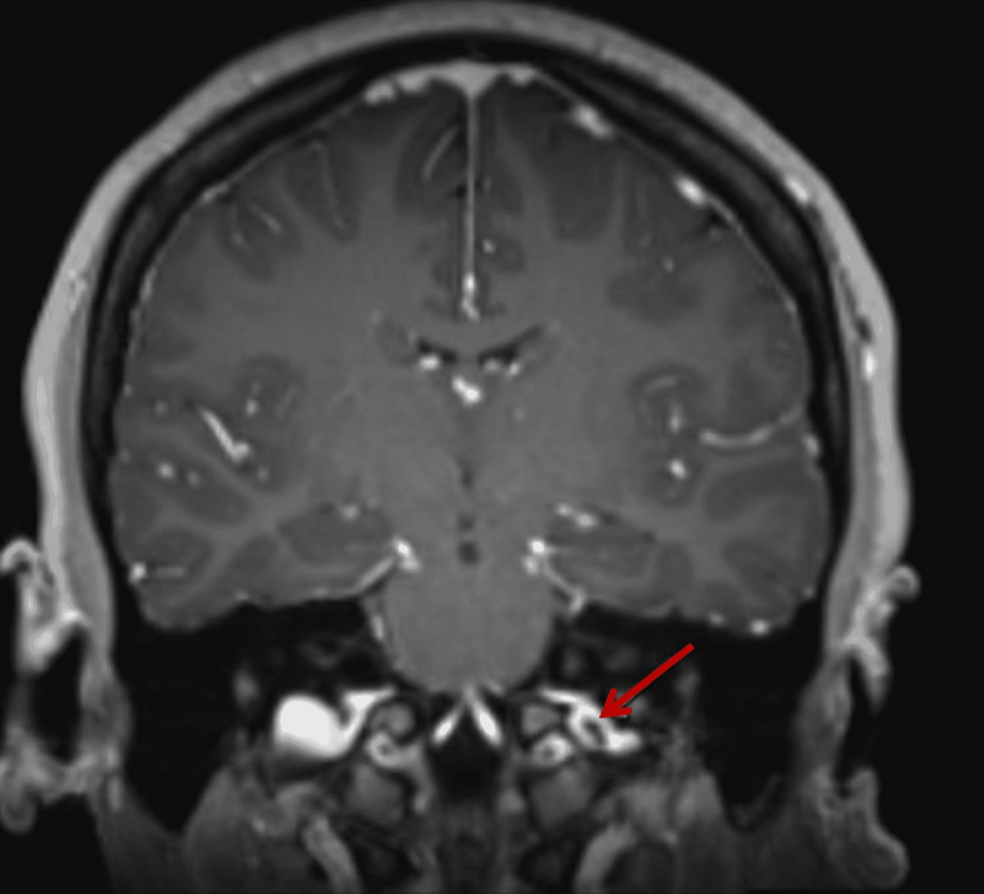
T-1 weighted coronal section MRI of the brain demonstrating a non-occlusive thrombus in the left IJV at the level of the jugular bulb at the skull base (red arrow)
